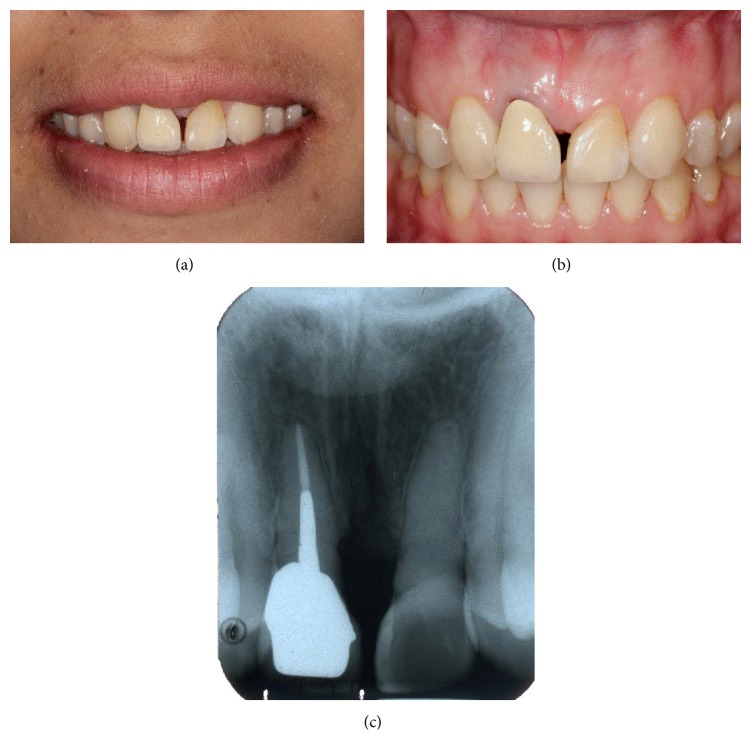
Pretreatment extra and intraoral photograph and periapical radiograph. (a) Smile photograph. (b) Intraoral photograph (front view) showing a midline diastema. (c) Periapical radiograph of the lateral incisors.
